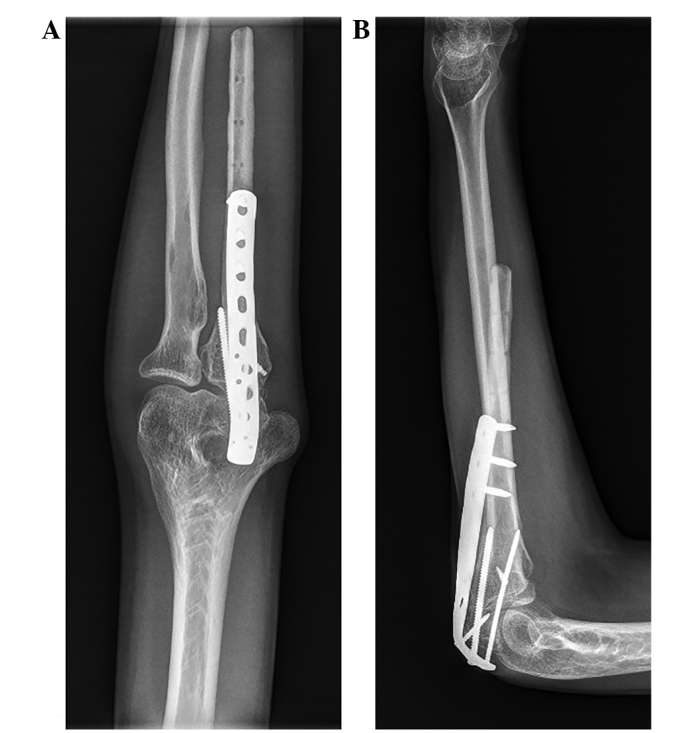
(A) Anteroposterior and (B) lateral radiographs 2 years after surgery. Restoration of the proximal ulnar anatomy occurred without severe problems.
